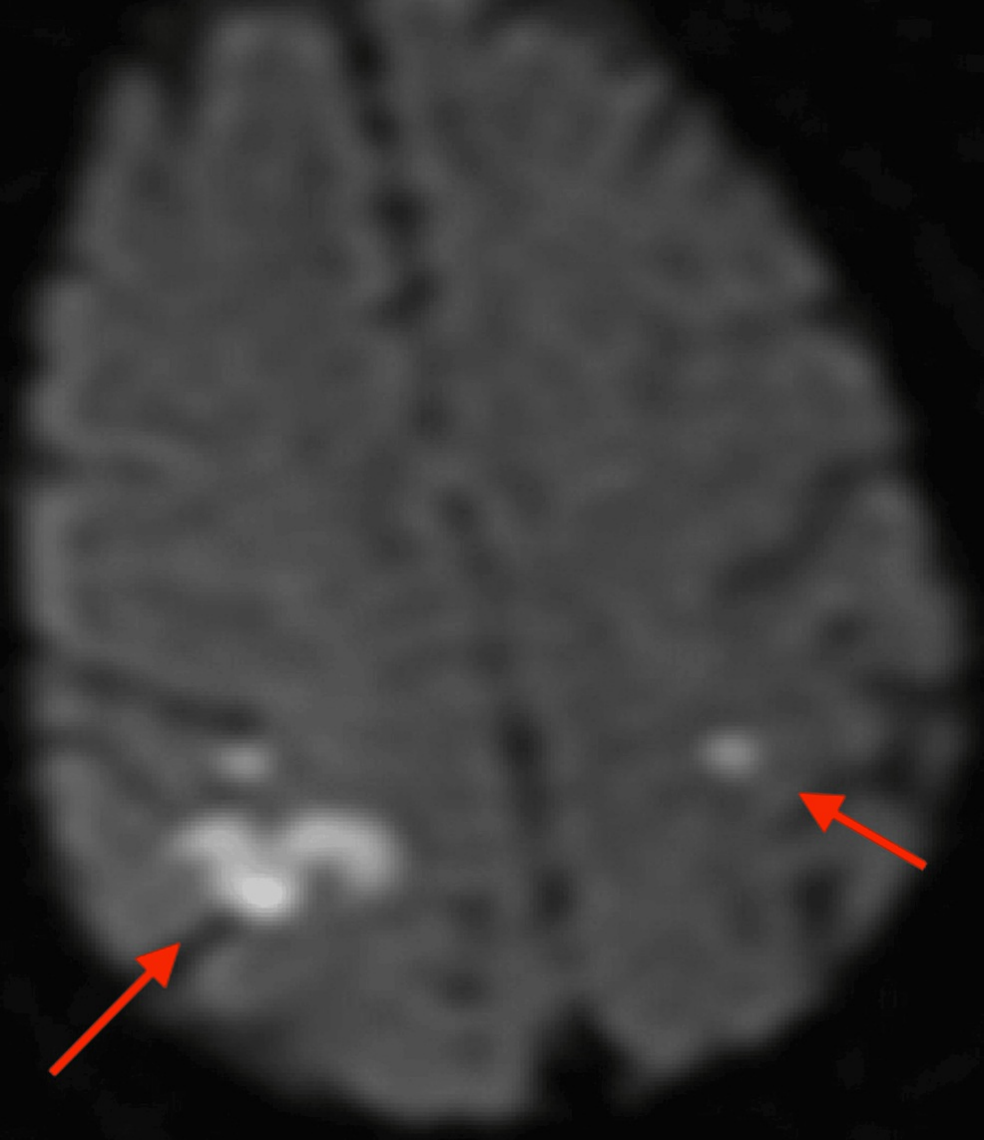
Brain MRI DWI axial Acute and subacute lacunar infarcts involving the high left posterior frontal and parietal subcortical white matter. DWI: Diffusion-weighted imaging
